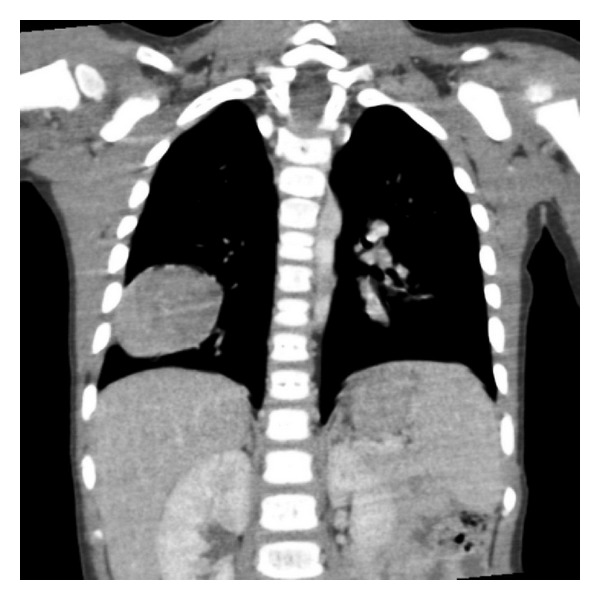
CT chest: right lung lower lobe peripheral mass.
